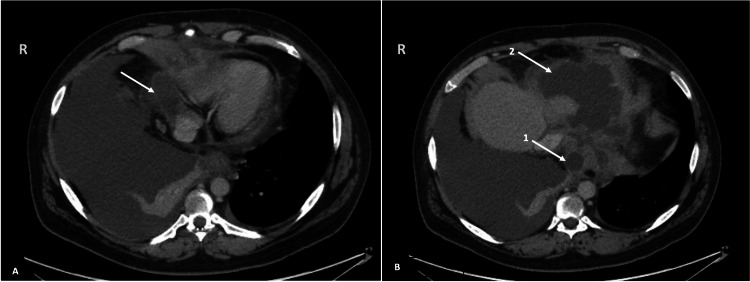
CT of the abdomen and pelvis showing a cardiophrenic collection which abuts the right heart (arrow) (A) and two thoracic fluid collections (B). The first collection is at the gastroesophageal junction and the diaphragmatic hiatus (arrow 1), and the second is a loculated gastrohepatic collection (arrow 2). CT: computed tomography
